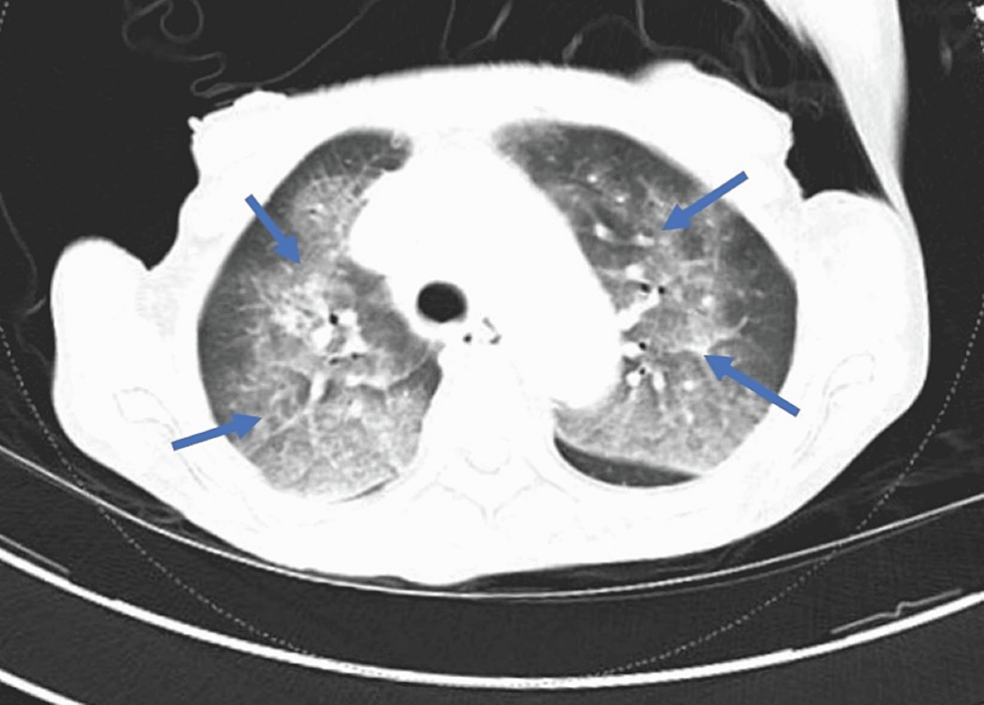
CT chest showing septal thickenings and patchy acinar opacities in the perihilar and central areas of both lungs
